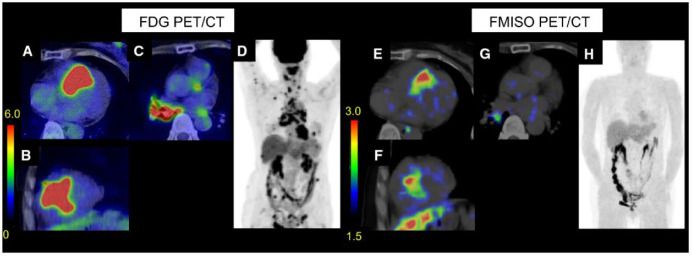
Example of ^18^F-FDG PET/CT and ^18^F-FMISO PET/CT images in a patient with cardiac sarcoidosis. Transverse, short-axis, and maximum intensity projection (MIP) images are provided. Significant abnormal cardiac ^18^F-FDG uptake was seen, as well as abnormal uptake in lymph nodes in the neck, mediastinum, hilum, abdomen, and inguinal lymph nodes (A–D). ^18^F-FMISO showed corresponding abnormal cardiac uptake, as well as abnormal uptake in a right hilar lymph node (E–H). Accessed on 11 July 2021. Modified and reprinted with permission from ref. [86]. Copyright 2021 Furuya, S. et al.
